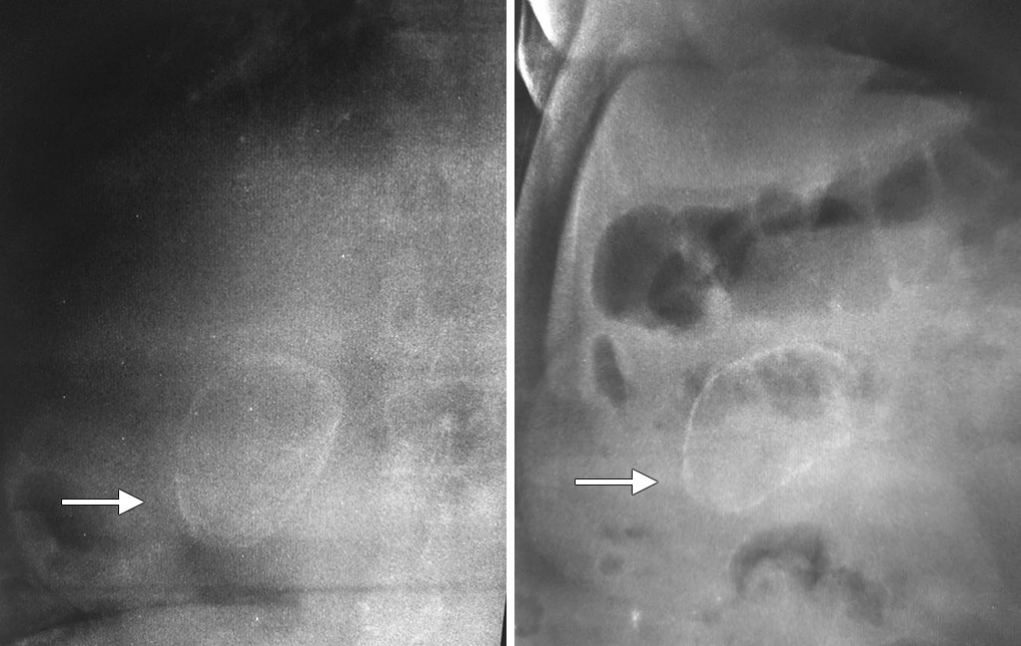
Plain X-ray abdomen showing calcified gall bladder
